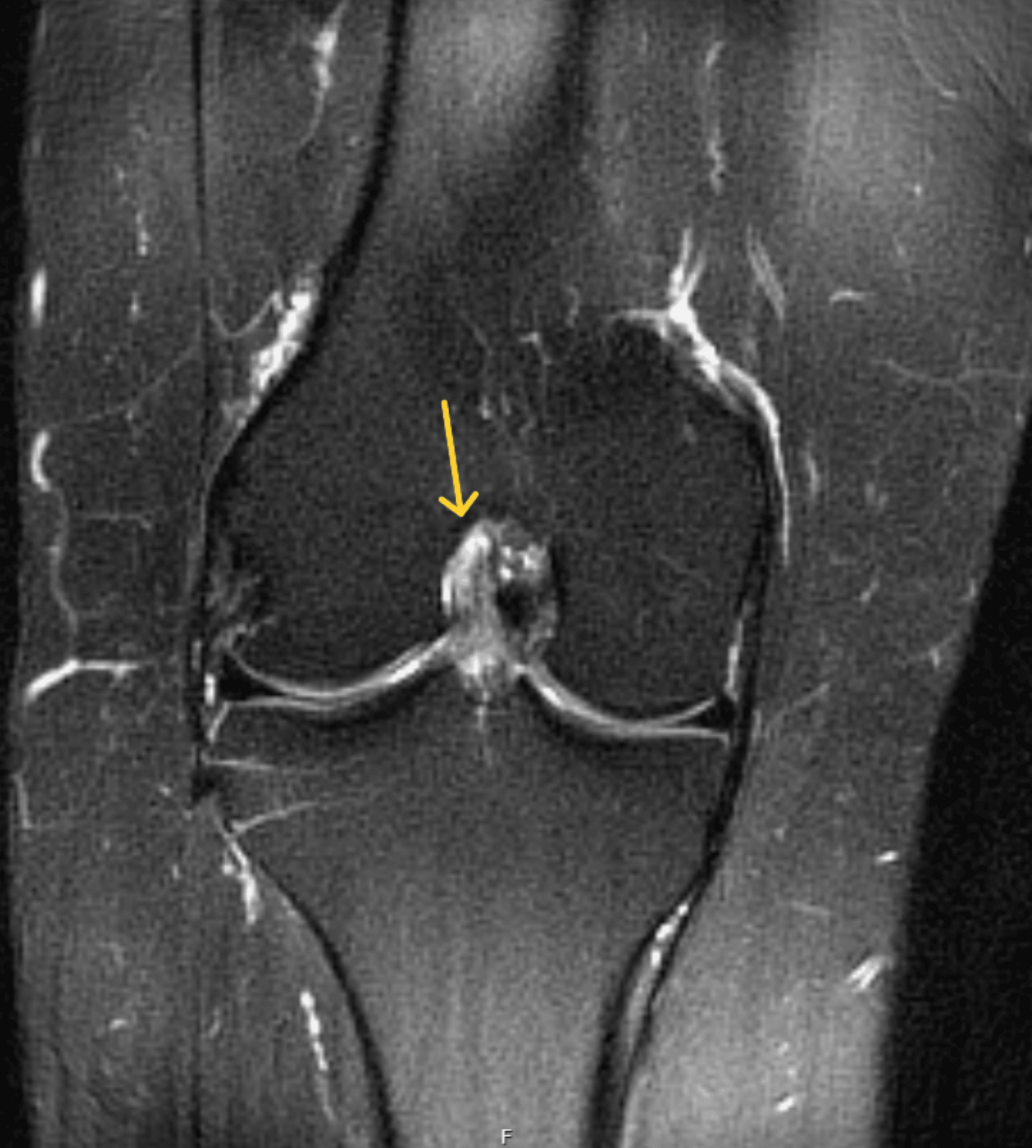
Coronal T2-weighted fat-saturated MRI of the right knee demonstrating an ACL tear (yellow arrow) ACL: anterior cruciate ligament
